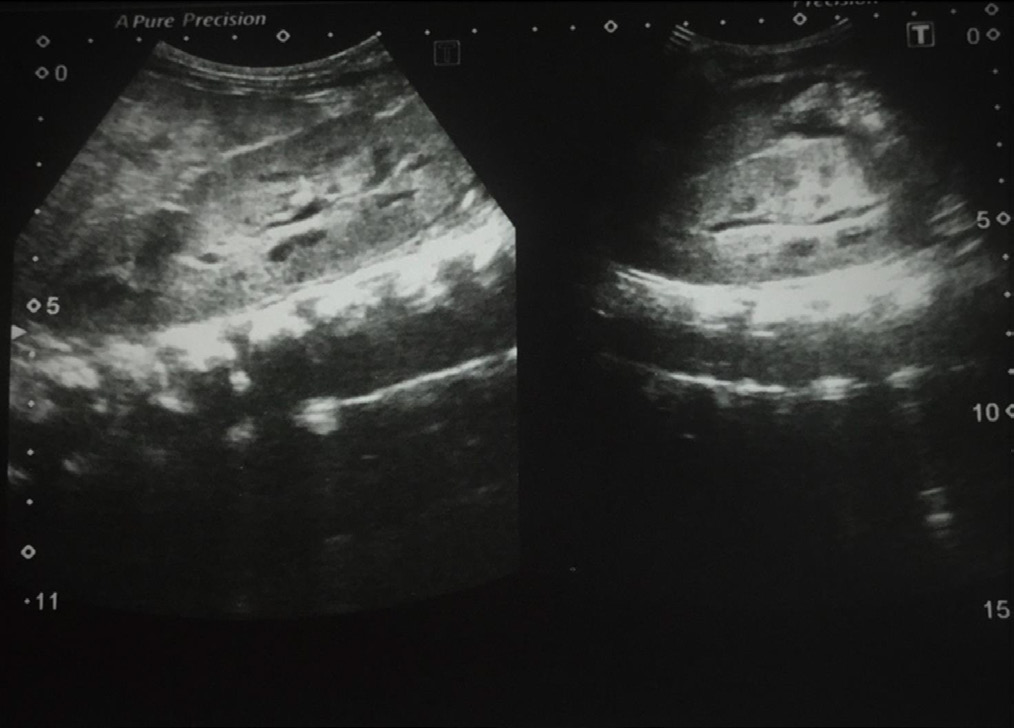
Ultrasound abdomen demonstrates bilateral enlarged echogenic kidneys.
